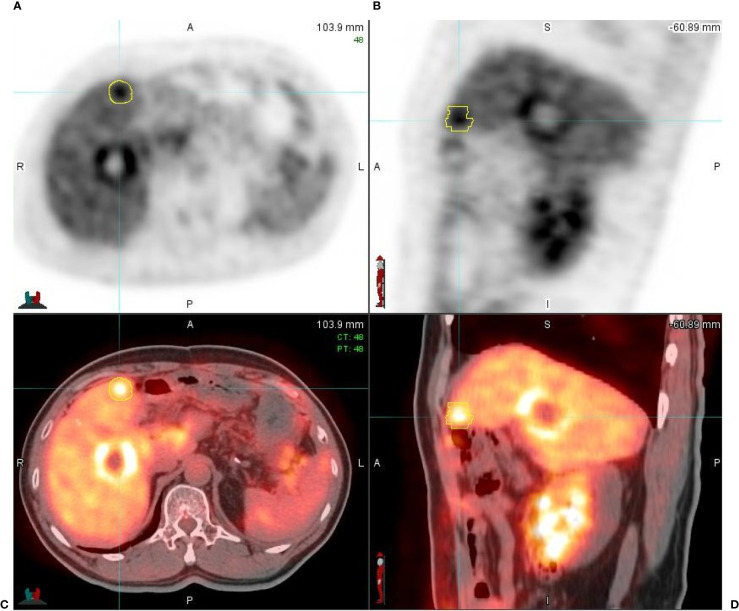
A 65-year-old male patient with hepatic metastasis from a primary rectal cancer. (A) Axial respiratory gated PET image (PET_0% sorted) shows two liver lesions with an increased uptake of FDG; one of the liver lesions is contoured (in yellow), measuring 2.5 cm × 2.3 cm in diameter with SUV_max_ = 9.24, conforming to liver metastasis. (B) Sagittal respiratory gated PET image (PET_0% sorted); one of the lesions is contoured (in yellow), measuring 2.8 cm in diameter with SUV_max_ = 9.24. (C, D) The respiratory gated PET axial and sagittal images fused with respiratory gated CT images (PET/CT_0% sorted); the high FDG uptake area is presented in thermal color and CT images are in gray scale.
